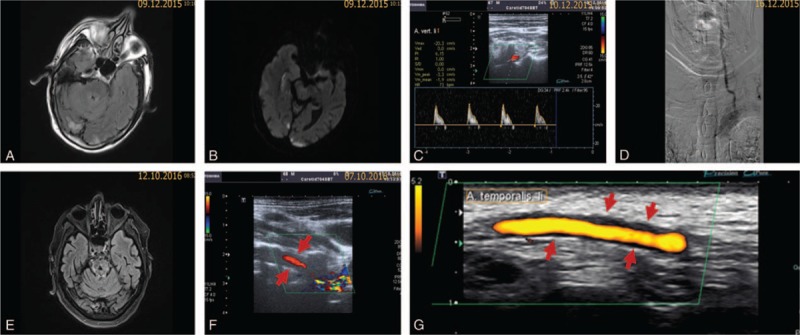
Fluid-attenuated inversion recovery (A) and Diffusion-weighted imaging (B) in 12/2015 showed multiple small acute infarctions in the vertebrobasilar territory. Duplex ultrasound of the left vertebral artery showed occlusion signal denoting distal occlusion (C), which was confirmed in the DSA (D). FLAIR in 10/2016 showed a lacunar cavity in the pons (E), which was not present in the initial MRI images. Duplex ultrasound in 10/2016 showed recanalization and “halo” sign (red arrows) around the left vertebral artery (F). Ultrasound of the left superficial temporal artery in 10/2016 showed “halo” sign (red arrows) supporting the diagnosis (G).
